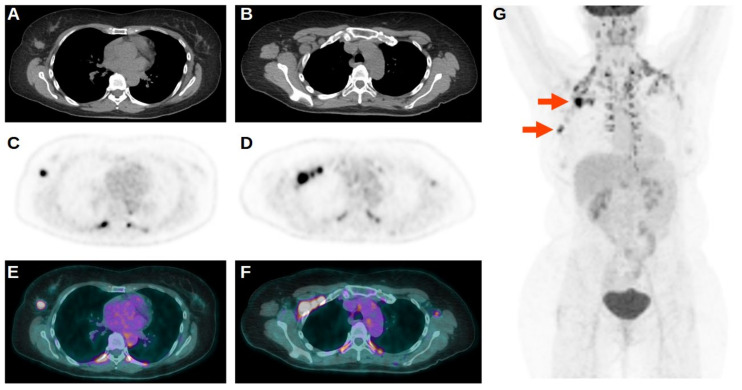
Axial CT (A,B), axial PET (C,D), axial fused PET/CT, (E,F) and maximum intensity projection (MIP) (G) of a [^18^F]FDG PET/CT scan performed in a patient positive for BRCA1 mutation, demonstrating intense uptake of tracer on primary right BC and concomitant multiple focal uptakes on metastatic axillary and retropectoral nodes (red arrows on MIP). Brown adipose tissue activation was also reported.
